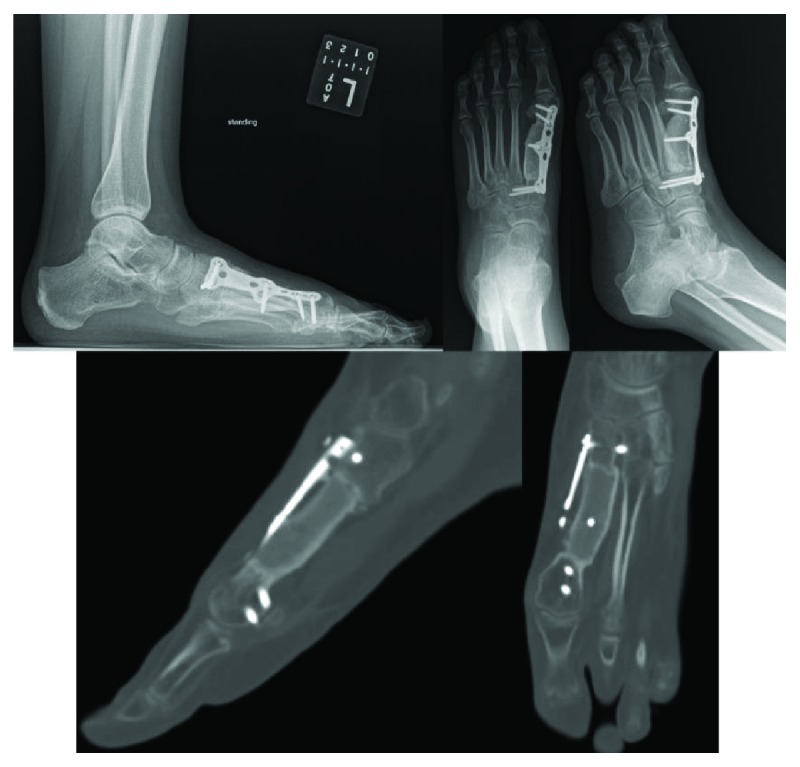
Nine-month postoperative plain radiographs and CT scan demonstrating osseous bridging at both the proximal and distal graft sites with no signs of tumor recurrence.
